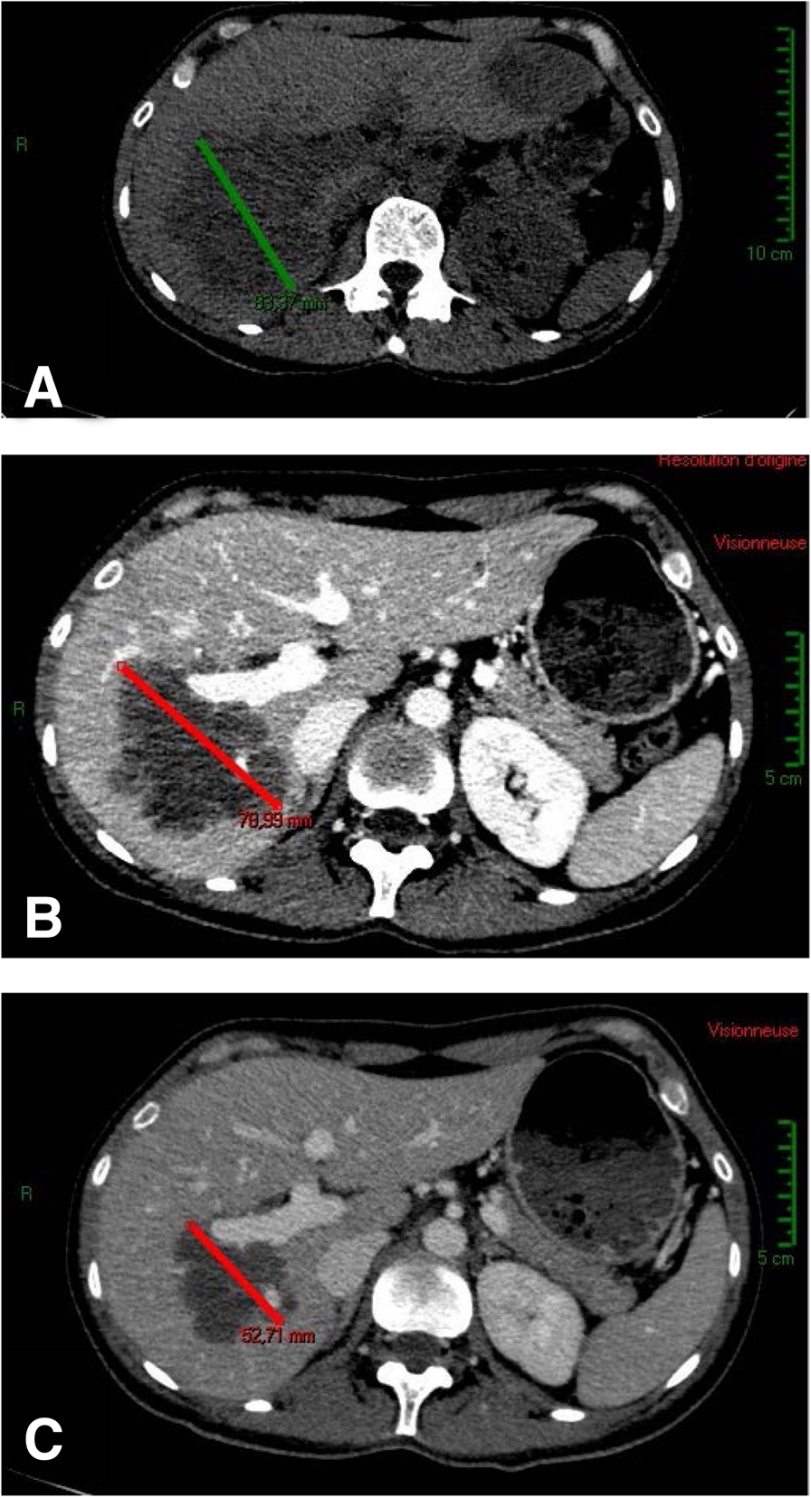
Before and after the treatment. CT scans showing hepatic metastases before the treatment (a), and the tumor size reduction after 3 cycles of chemotherapy (b) and after 6 cycles (c)
